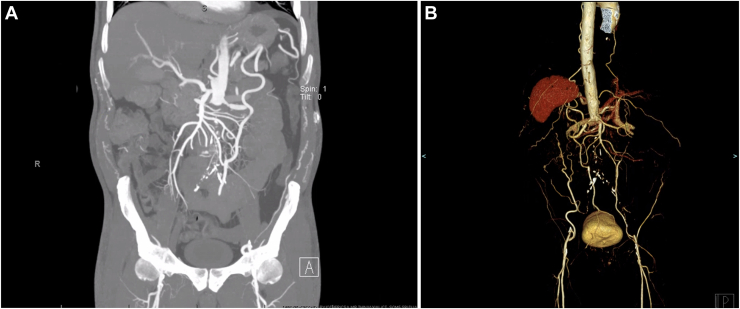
Coronal computed tomography angiogram (A) and 3-dimensional volume rendered image (B) of the abdomen demonstrating complete occlusion of the infrarenal abdominal aorta distal to the origin of the renal arteries. Extensive collateral vessels from the internal thoracic and intercostal arteries are visualized supplying the iliac circulation bilaterally.
